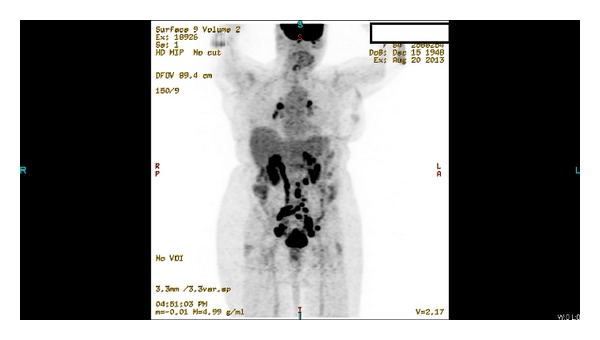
PET CT scan showing cervical mass, consistent with carcinoma, with diffuse lymphadenopathy throughout the abdomen, pelvis, and the hila of both lungs.
